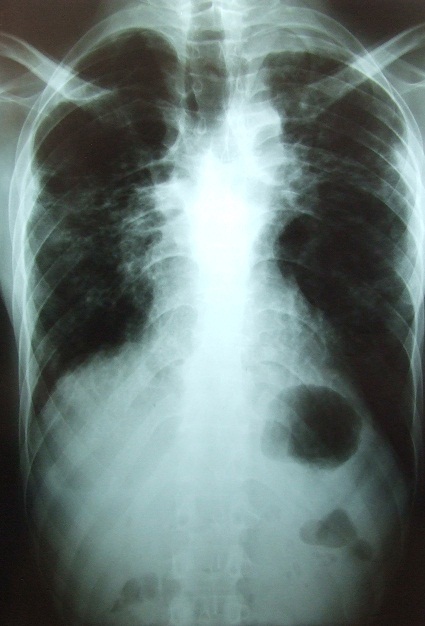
Radiographie de contrôle après ablation du drain
